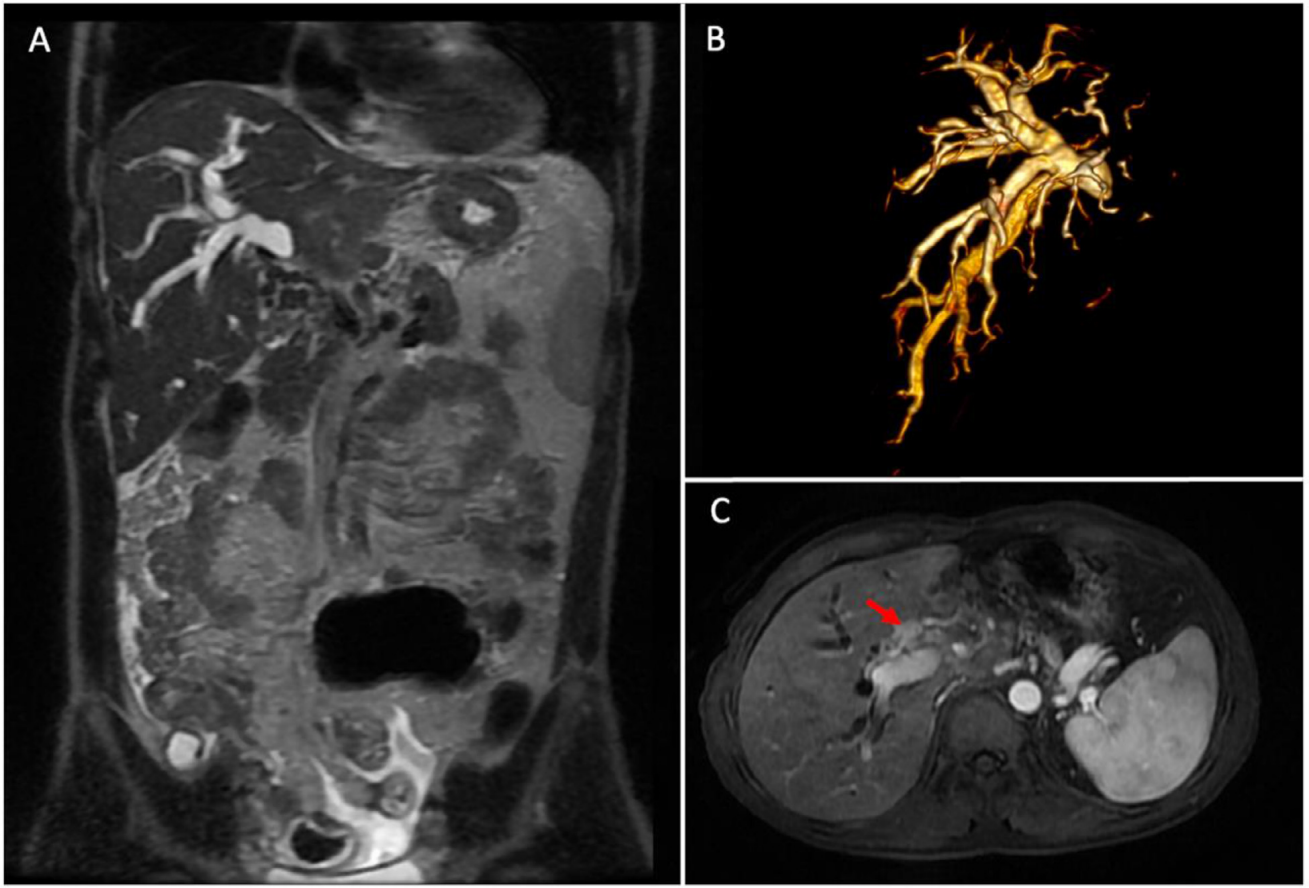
Abdominal MRI; (A) T2 FS sequence coronal view showed the dilatation followed by an abrupt termination of right intrahepatic bile duct; (B) 3D MRCP clearly showed the dilatation of right hepatic bile duct; (C) T1 FS contrast showed the contrast-enhanced mass (red arrow) that obstruct the right intrahepatic bile duct. © Department of Radiology, Dr. Soetomo General Academic Hospital, Medical Faculty of Universitas Airlangga, Surabaya, Indonesia (with permission).
